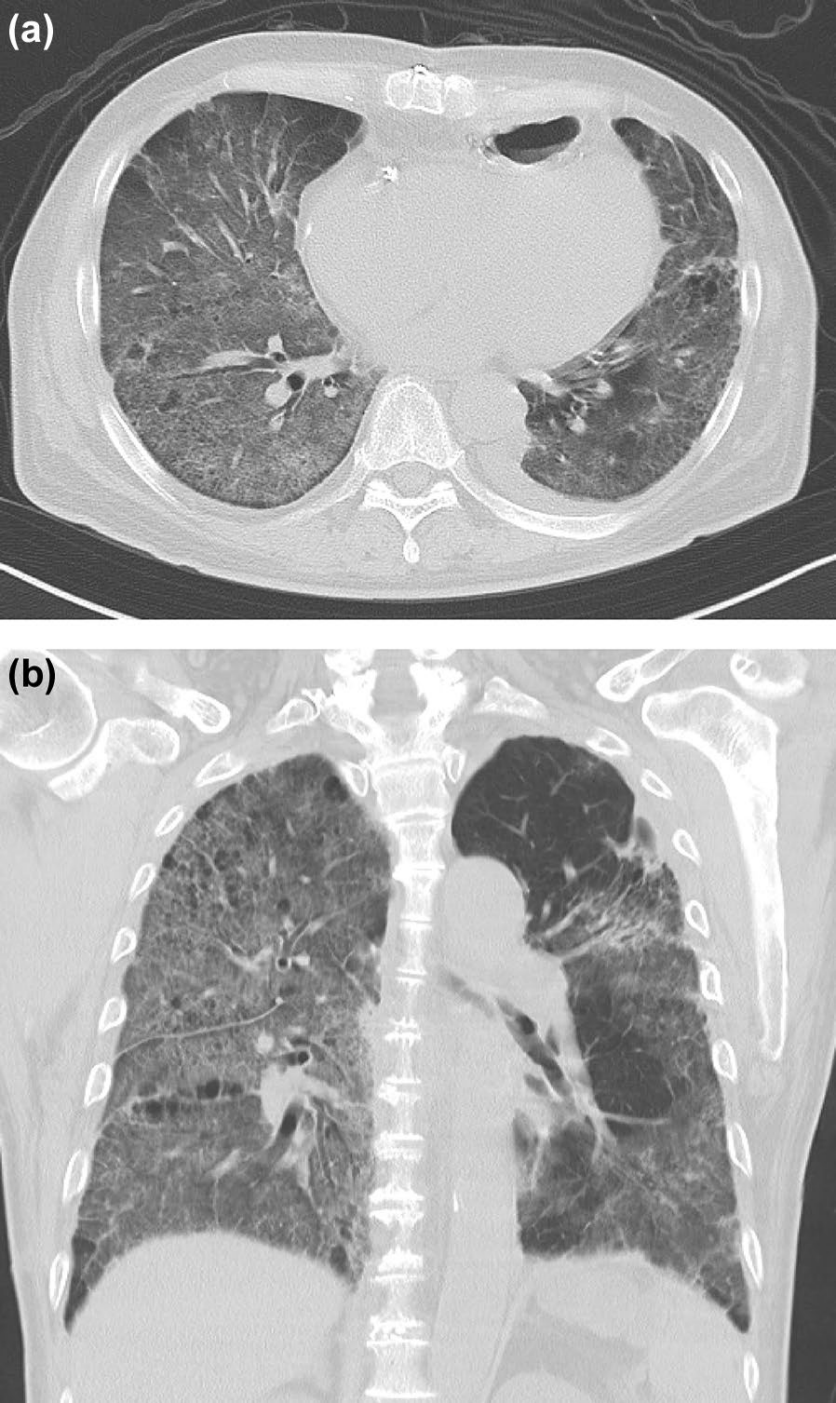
CT showing extensive interstitial shadows in both lungs following worsening respiratory condition after hospitalization in Case 2. a: coronal. b: axial. CT, computed tomography
